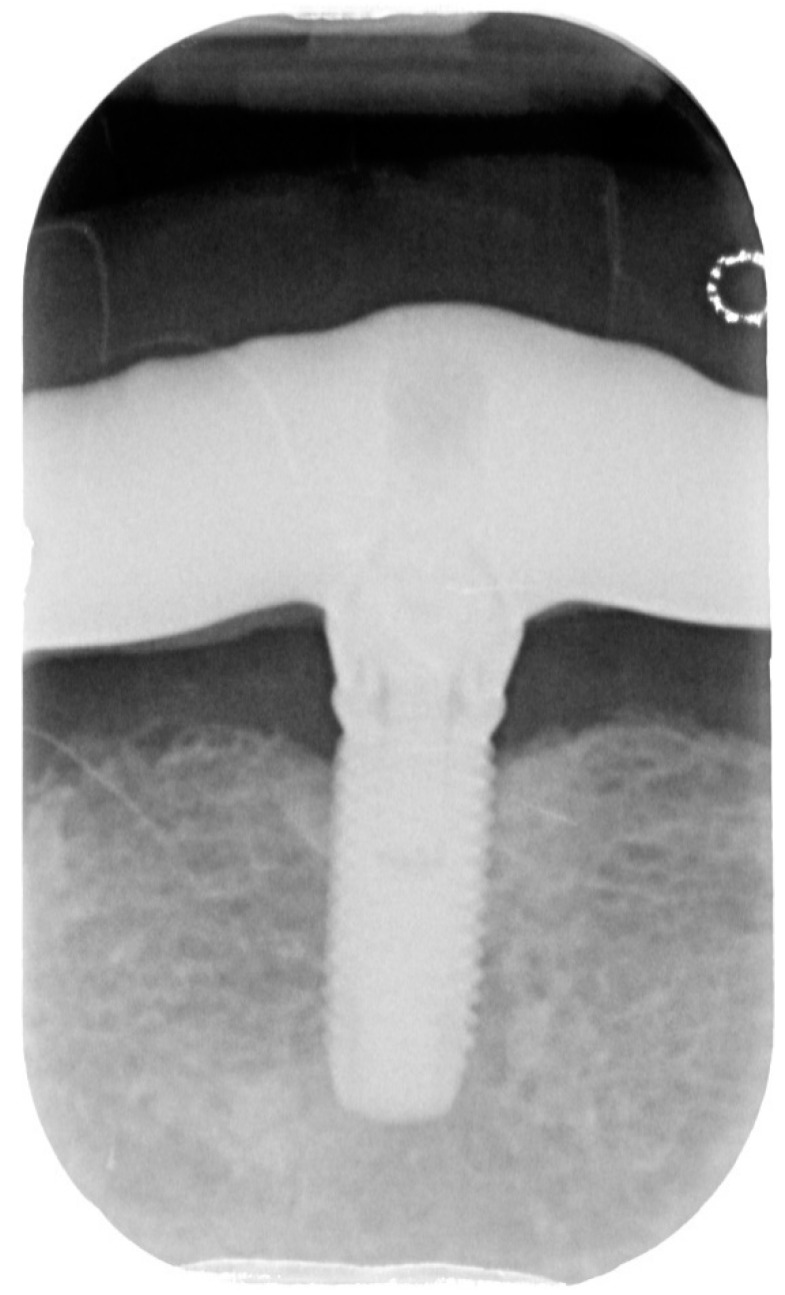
X-ray of dental implant area after treatment.
